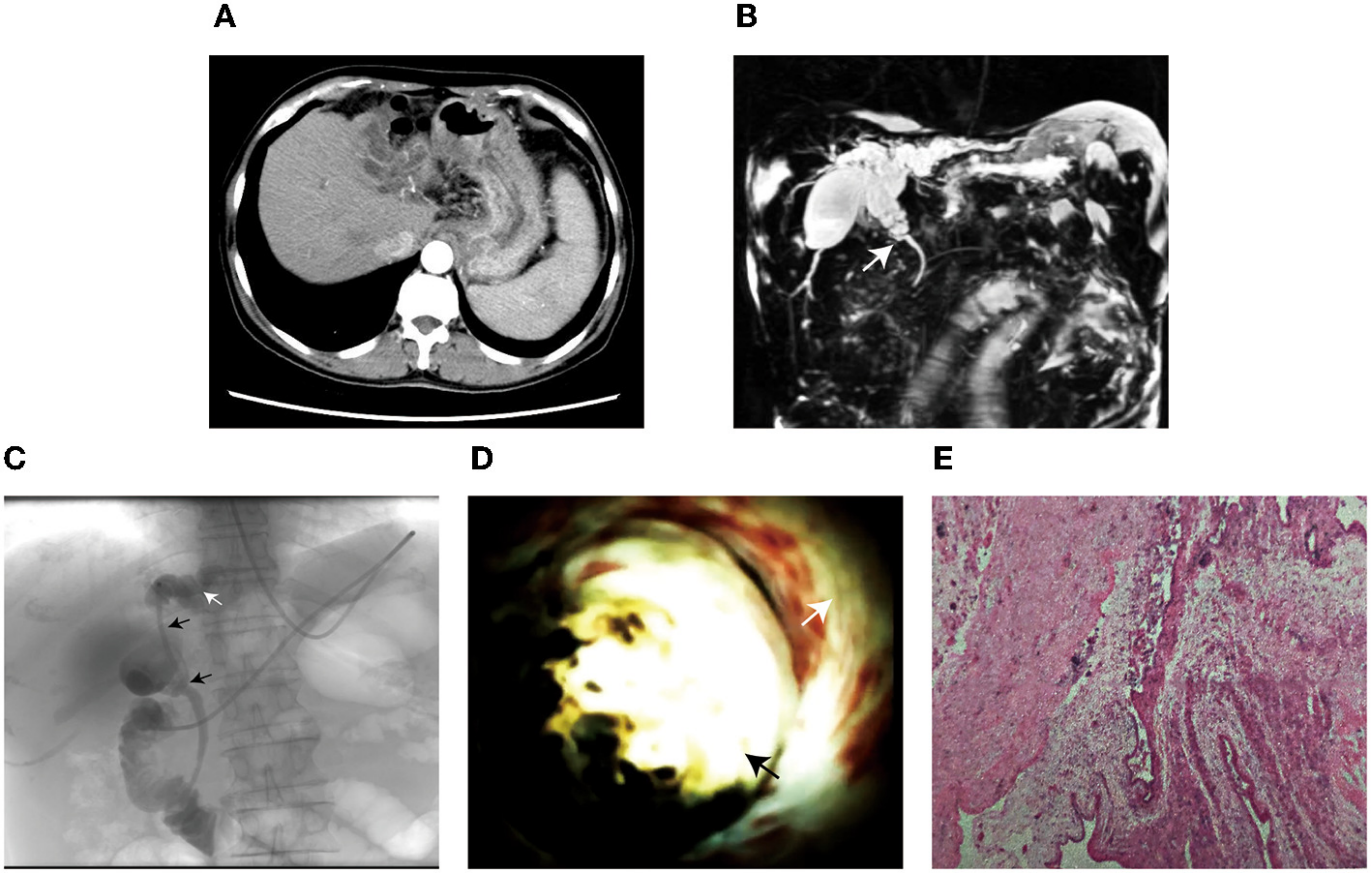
(A) Enhanced CT showed the dilation of intrahepatic bile duct. (B) MRCP showed the common bile duct stricture in the head of the pancreas (white arrow), additional soft tissue shadows were not seen at the surrounding regions and the upstream common bile duct and intrahepatic bile duct dilated significantly. (C) ERCP indicated that biliary stricture was at the upper common bile duct and hilar bile duct (black arrows) and left intrahepatic bile duct dilated significantly. (D) SpyGlass showed that an oval space-occupying lesion was seen at the upper segment of the common bile duct (black arrow). The biliary wall (white arrow) was smooth. The lesion filled the bile duct lumen, resulting in bile duct obstruction. The surface of this lesion was smooth, without dilated and tortuous blood vessels. (E) Postoperative pathological examination revealed secretory biliary cystadenoma.
